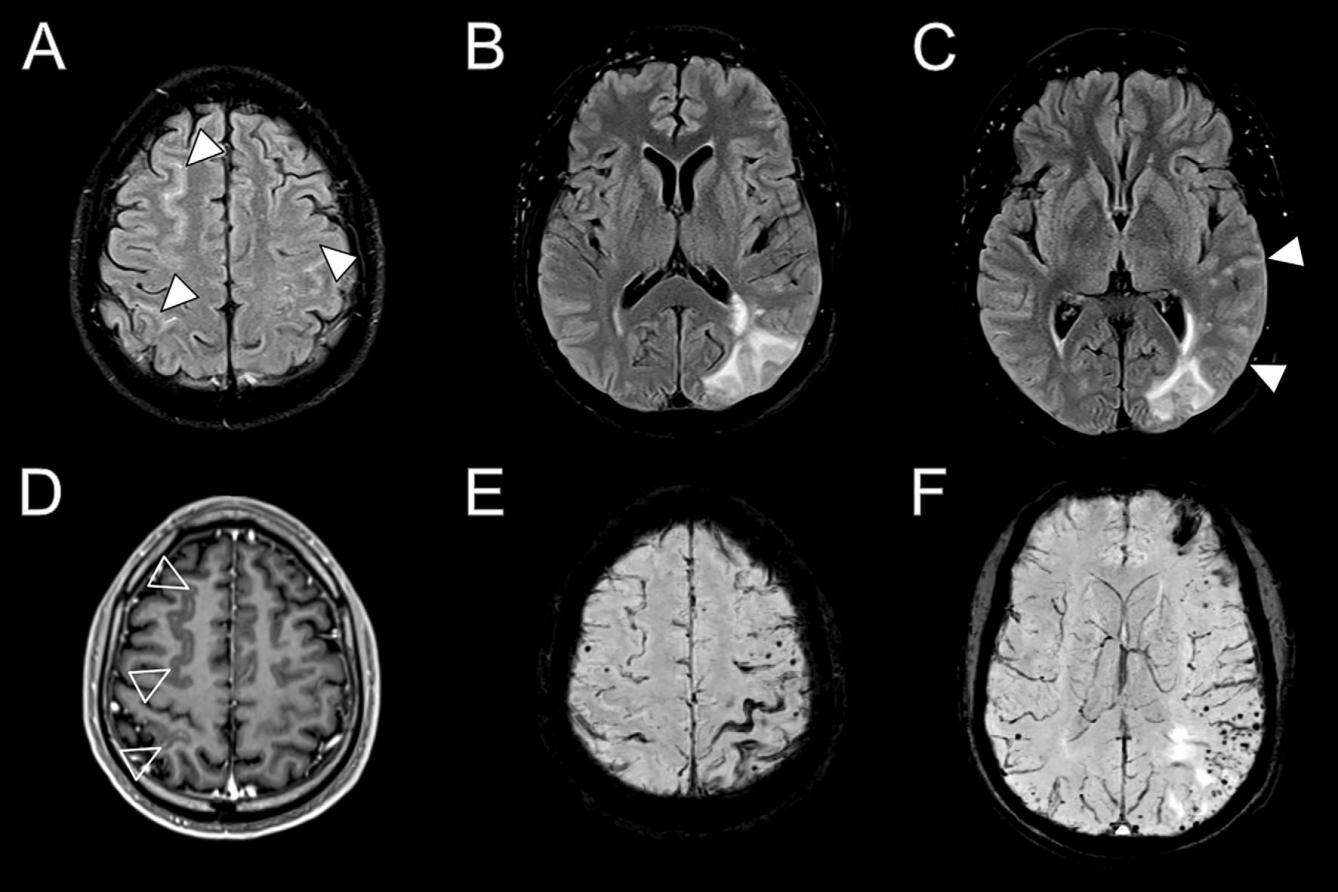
Representative magnetic resonance imaging biomarkers of cerebral amyloid angiopathy‐related inflammation. (A–C) Fluid attenuated inversion recovery hyperintensity imaging of the same patient demonstrated (A) convexity sulcal hyperintensities (white arrows), (B, C) left parieto‐occipital white matter hyperintensities, and (C) gyral swelling (white arrows). (D) Contrast‐enhanced T1‐weighted image showed leptomeningeal enhancement (arrowheads). (E, F) Susceptibility‐weighted imaging showed extensive superficial siderosis that was most apparent in the left central sulcus and over the (E) left frontal pole, and (F) numerous lobar microhemorrhages.
